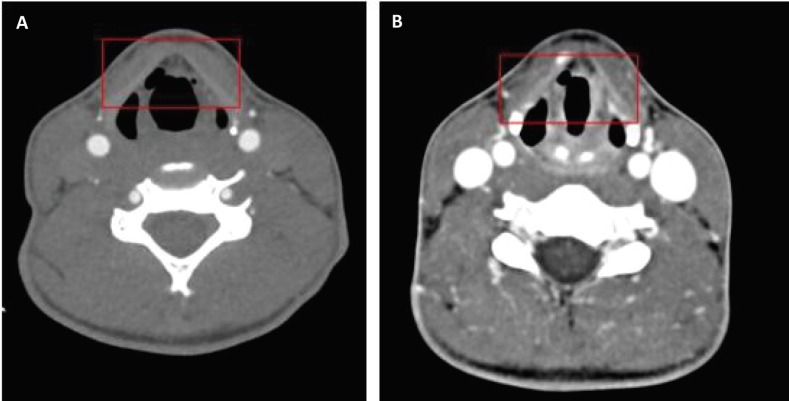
Axial Ct-scan of the neck demonstrating bilateral laryngoceles in the pre-epiglottic space with the right laryngocele larger than the left (A); axial CT-scan of the neck on day 3, demonstrating resolution of left laryngocele with the right decreased in size since the initial presentation
